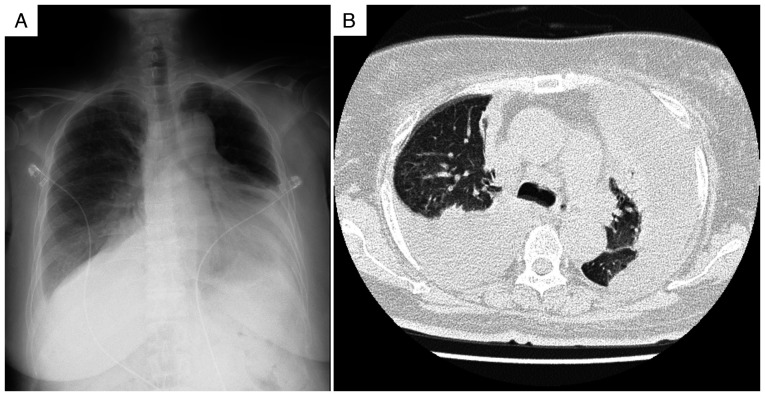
Radiological findings on hospital day 20, prior to the diagnosis of tuberculosis. (A) Chest radiograph showing persistent bilateral pleural effusions with notable progression of left-sided effusion. (B) Computed tomography revealing persistent bilateral pleural effusions despite optimal antihypertensive and diuretic therapy.
